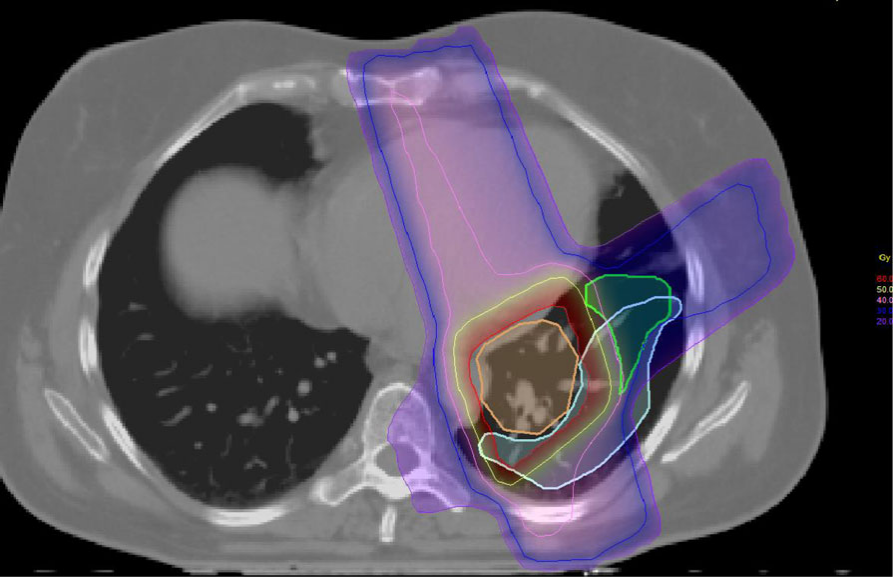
Example for the delineation of the RP volume. Two CT scans of the chest to confirm the clinical diagnosis and showing the maximum expansion of RP were fused with the treatment plan and both the pneumonitic lung areas at the time point of clinical appearance and at maximum expansion were delineated. In this example, the RP at the time point of clinical appearance (green contour) was located outside the 50 Gy isodose (yellow), mainly in lung areas with doses below 30 Gy. The larger RP volume at the time point of maximum expansion of RP (light blue contour) was also observed within the lung areas receiving more than 50 Gy (yellow 50 Gy isodose and red coloured the 60 Gy isodose) encompassing the bottom of the PTV (orange contour)
